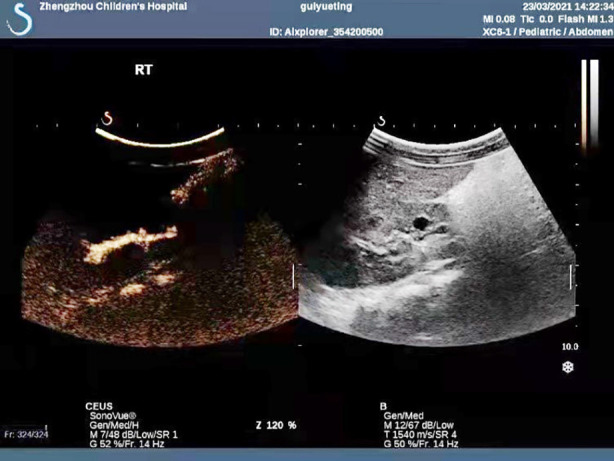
Image before contrast-enhanced cystosonography.
